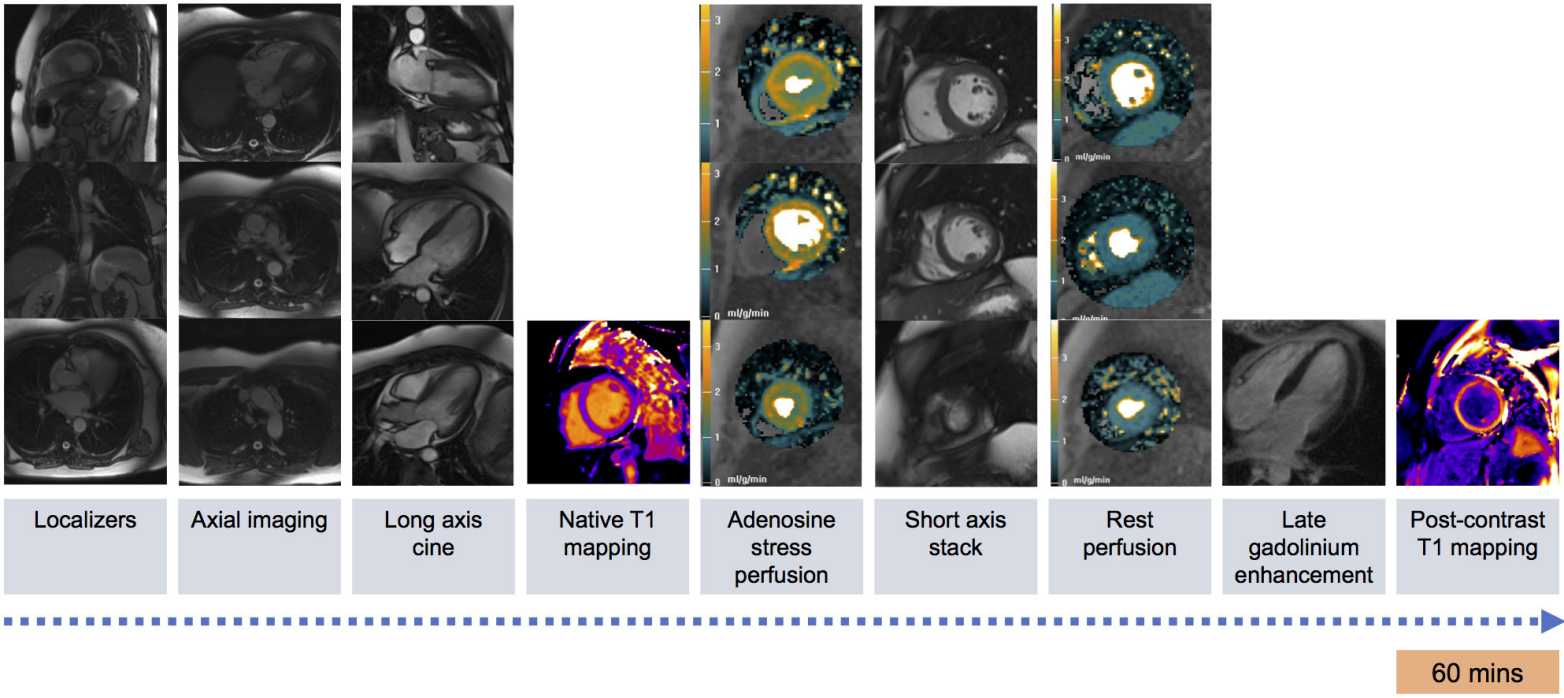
CorCMR multiparametric imaging protocol. CorCMR, Coronary Microvascular Angina Cardiac MRI.
